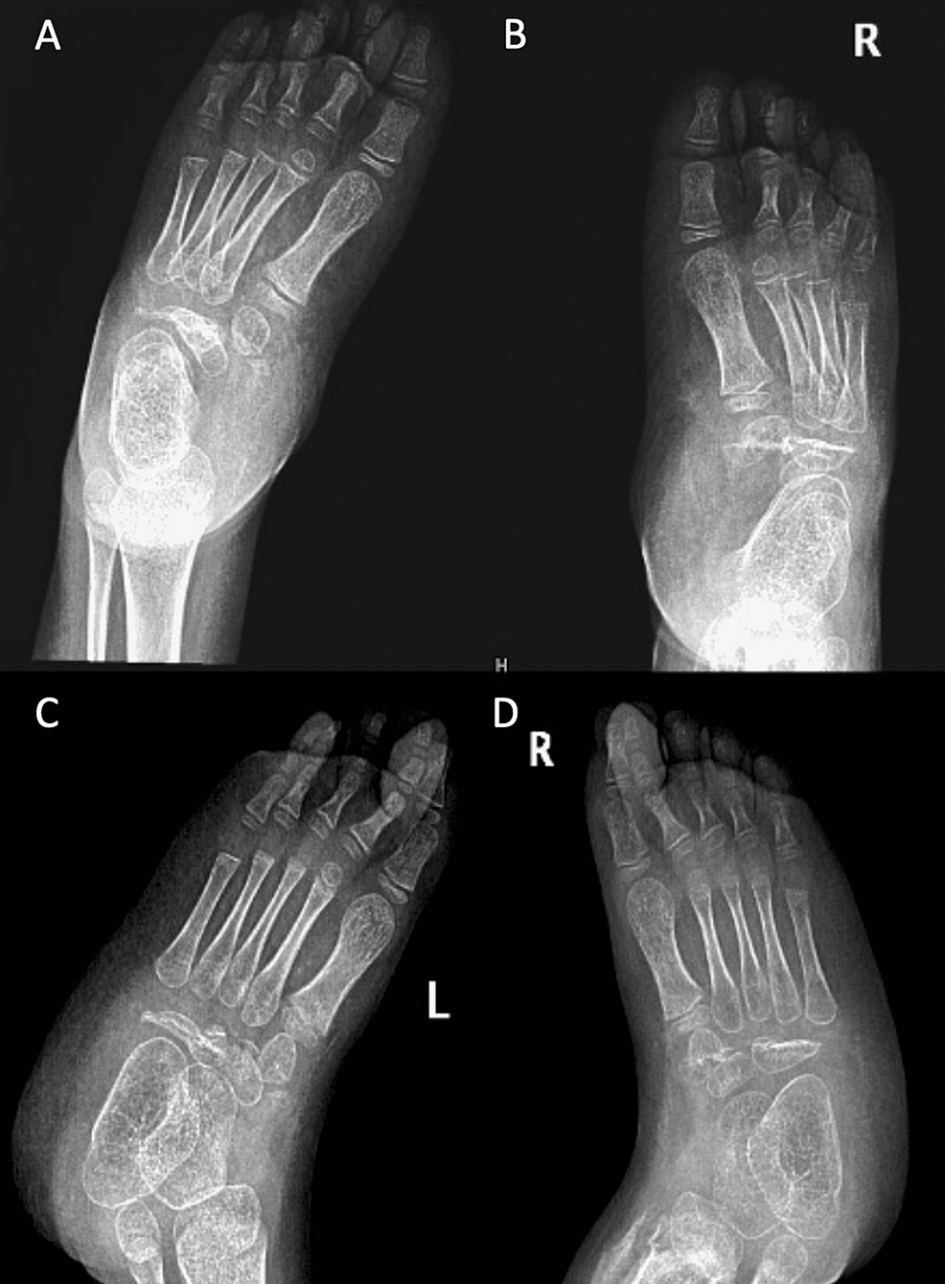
Three months postoperative x-ray images of Case 2 (A) Anteroposterior (AP) view of the left foot. (B) AP view of the right foot. (C) Oblique view of the left foot. (D) Oblique view of the right foot.
